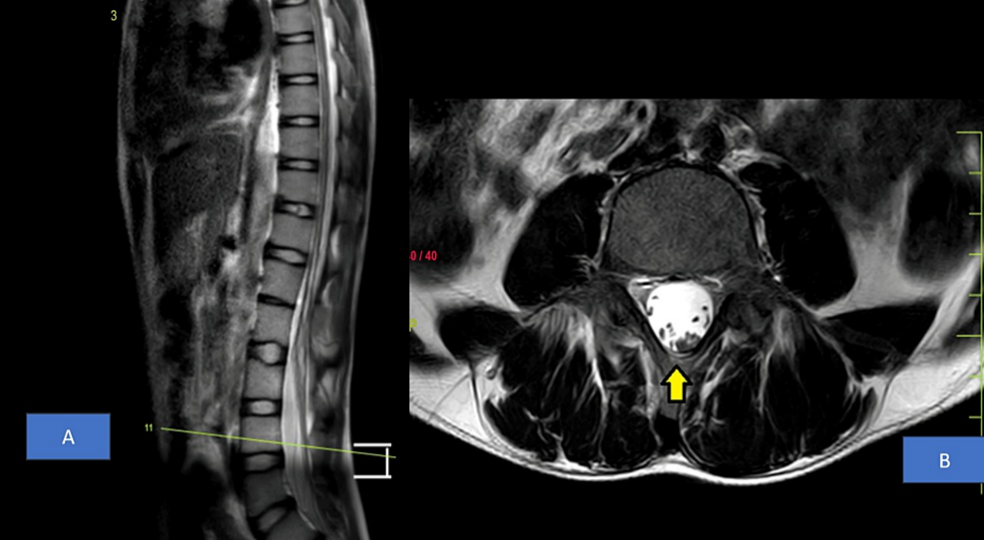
MRI Thoracolumbar Spine: A) T2-weighted sagittal section, B) T2-weighted axial section showing tethering of cauda equina nerve roots at the left lateral aspect of dural sac
